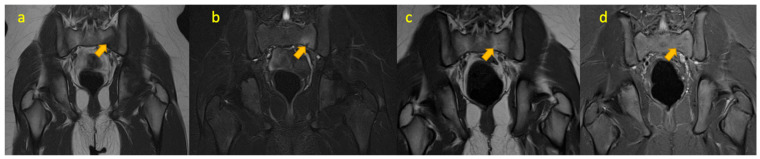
(a,b) Acute sacral insufficiency fracture due to vitamin D deficiency in a 12-year-old boy. Coronal T1-weighted and STIR MRI images demonstrate a left-sided sacral fracture surrounded by severe bone marrow oedema. image (c) coronal T1W image and image (d) coronal STIR images show post treatment imaging response with fatty marrow conversion.
